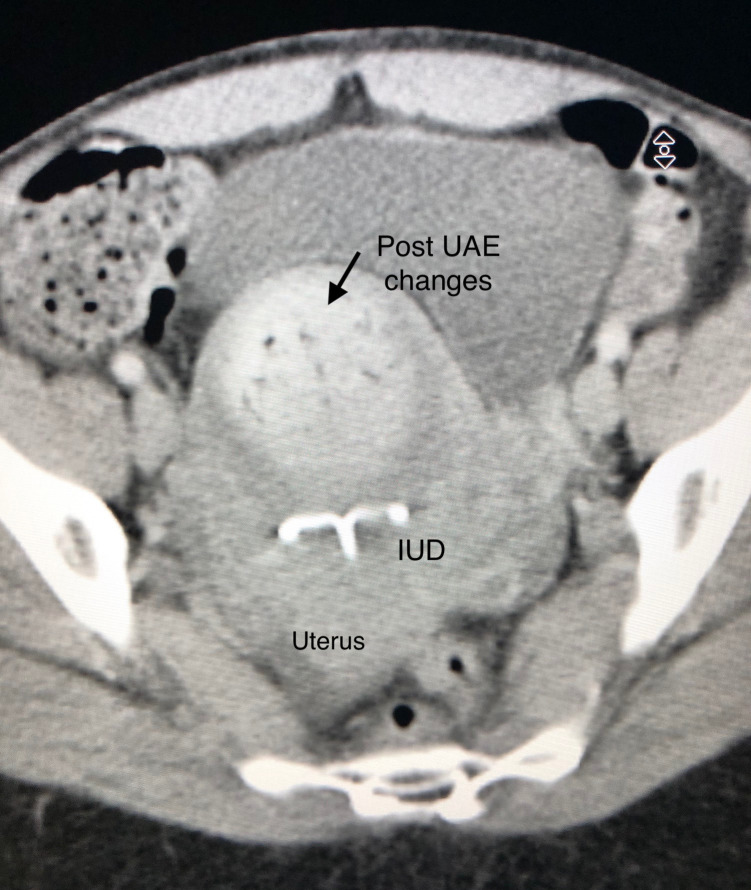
Siemens CT of the abdomen and pelvis with i.v. contrast in the portovenous phase demonstrates typical acute post-UAE changes within the fibroid. There is a small linear amount of gas and evidence of acute haemorrhagic infarction due to the high attenuation within the fibroids (arrow). The IUD is *in situ* and well within the endometrial cavity in proper position.
